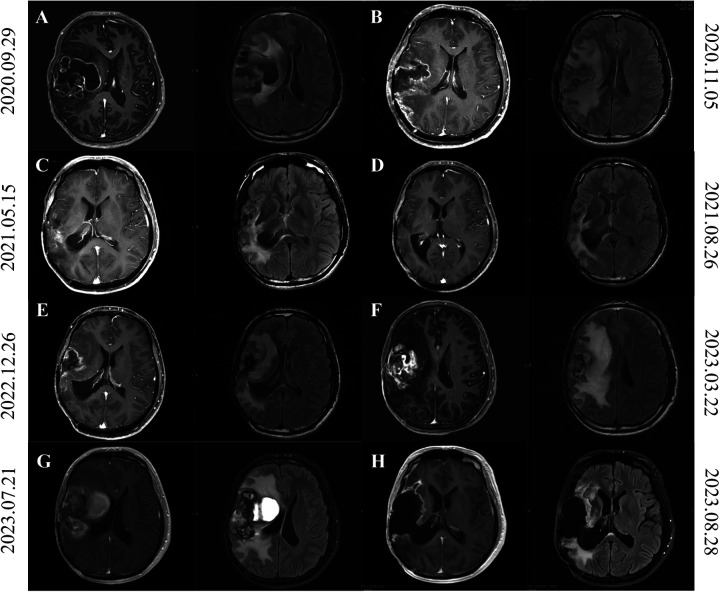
Brain MRI of patients from second tumor recurrence to third recurrence after surgery. (A) On September 29, 2020, MRI imaging demonstrated postoperative alterations in the right frontotemporal lobe, indicative of potential tumor recurrence. (B) MRI on November 5, 2020 showed a reduction in the size of lesions in the right frontotemporal lobe, a reduction in wall nodules, and a thickening of the meninges compared to MRI on September 29, 2020. (C,D) The cranial MRI on May 15, 2021 (C) and August 26, 2021 (D) was shown, showing that the tumor had progressed. (E,F) MRI of the head on March 22, 2023 (F), compared with the images on December 26, 2022 (E), showed slight enlargement of the right front parietotemporal lacunar after surgery and progressive deterioration of perifocal edema. (G) Figure shows the craniocerebral MRI findings in the presence of a tumor-derived hemorrhagic stroke. (H) This shows the postoperative craniocerebral MRI findings on August 23, 2023.
